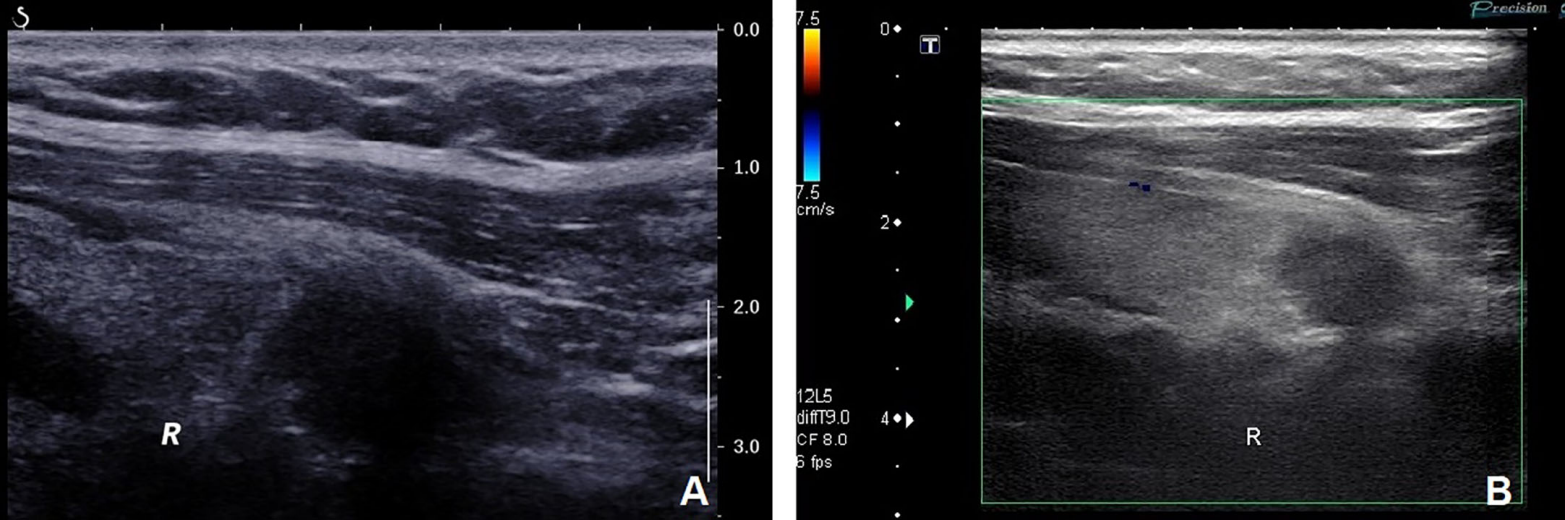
(A) Neck ultrasonography showed a solid hypoechoic nodule measuring 16mm×11mm×13mm in the middle-lower part of the right thyroid. The nodule exhibited irregular shape and unclear border but without calcification and linear hyperechoic patterns. The ratio of nodule longitudinal diameter to transverse diameter was less than 1. (B) Color Doppler sonography revealed scanty blood flow within the nodule.
